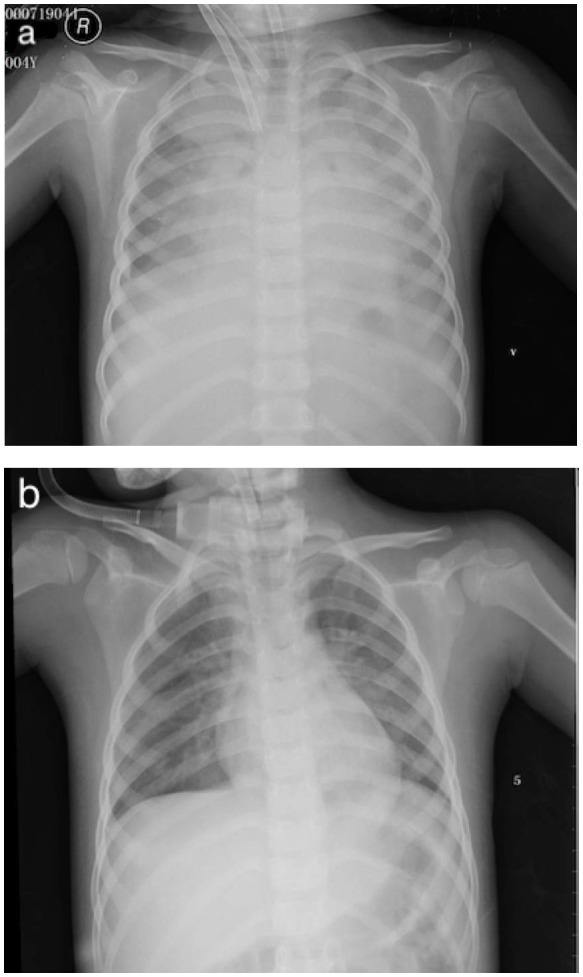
(a) V-A ECMO via neck cannulation with a 15-Fr cannula in the right atrium and a 12-Fr cannula in the right common carotid aorta. (b) Chest X-ray post-ECMO.
